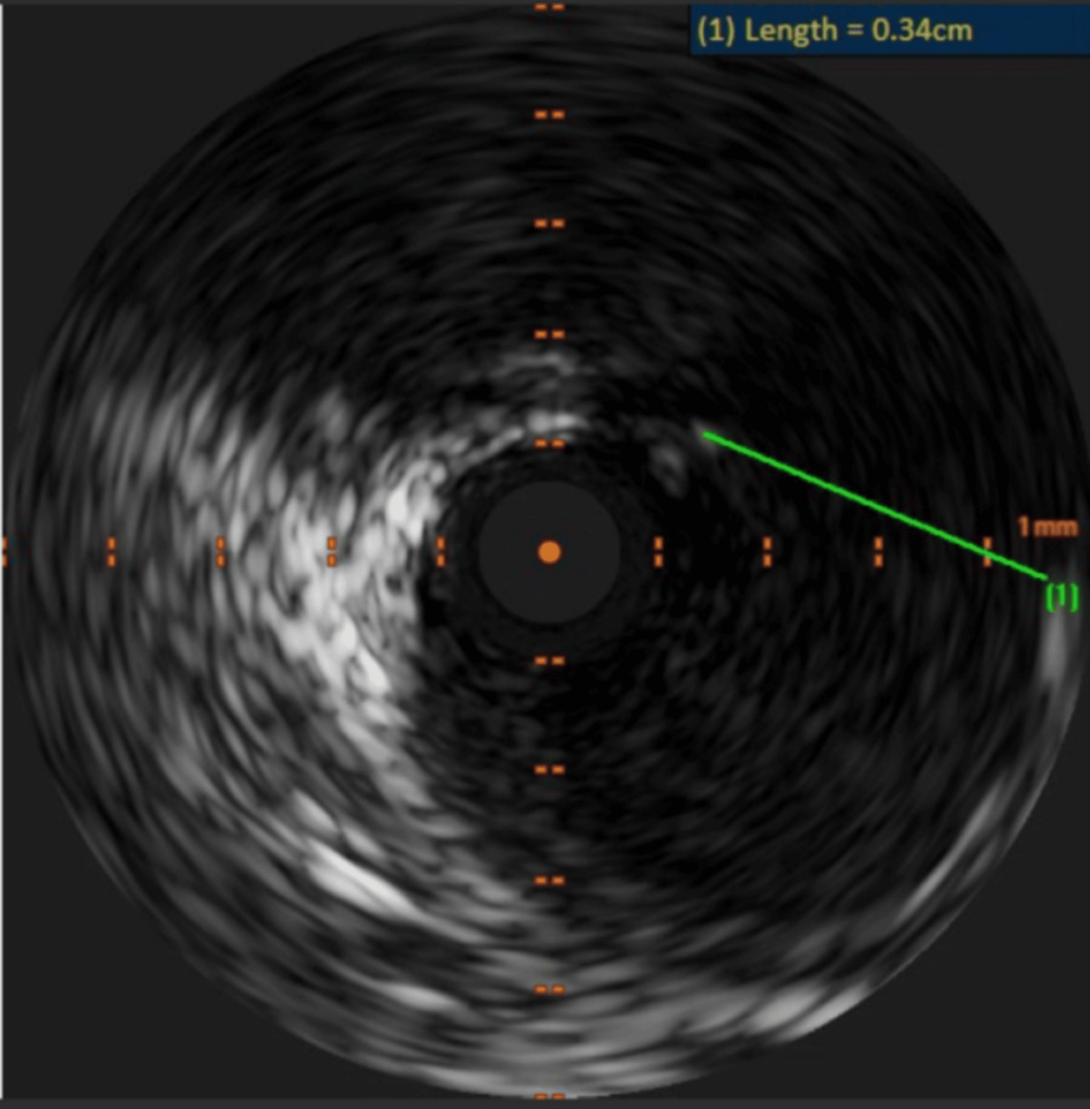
Intravascular ultrasound (IVUS) demonstrating the mouth of the aneurysm
